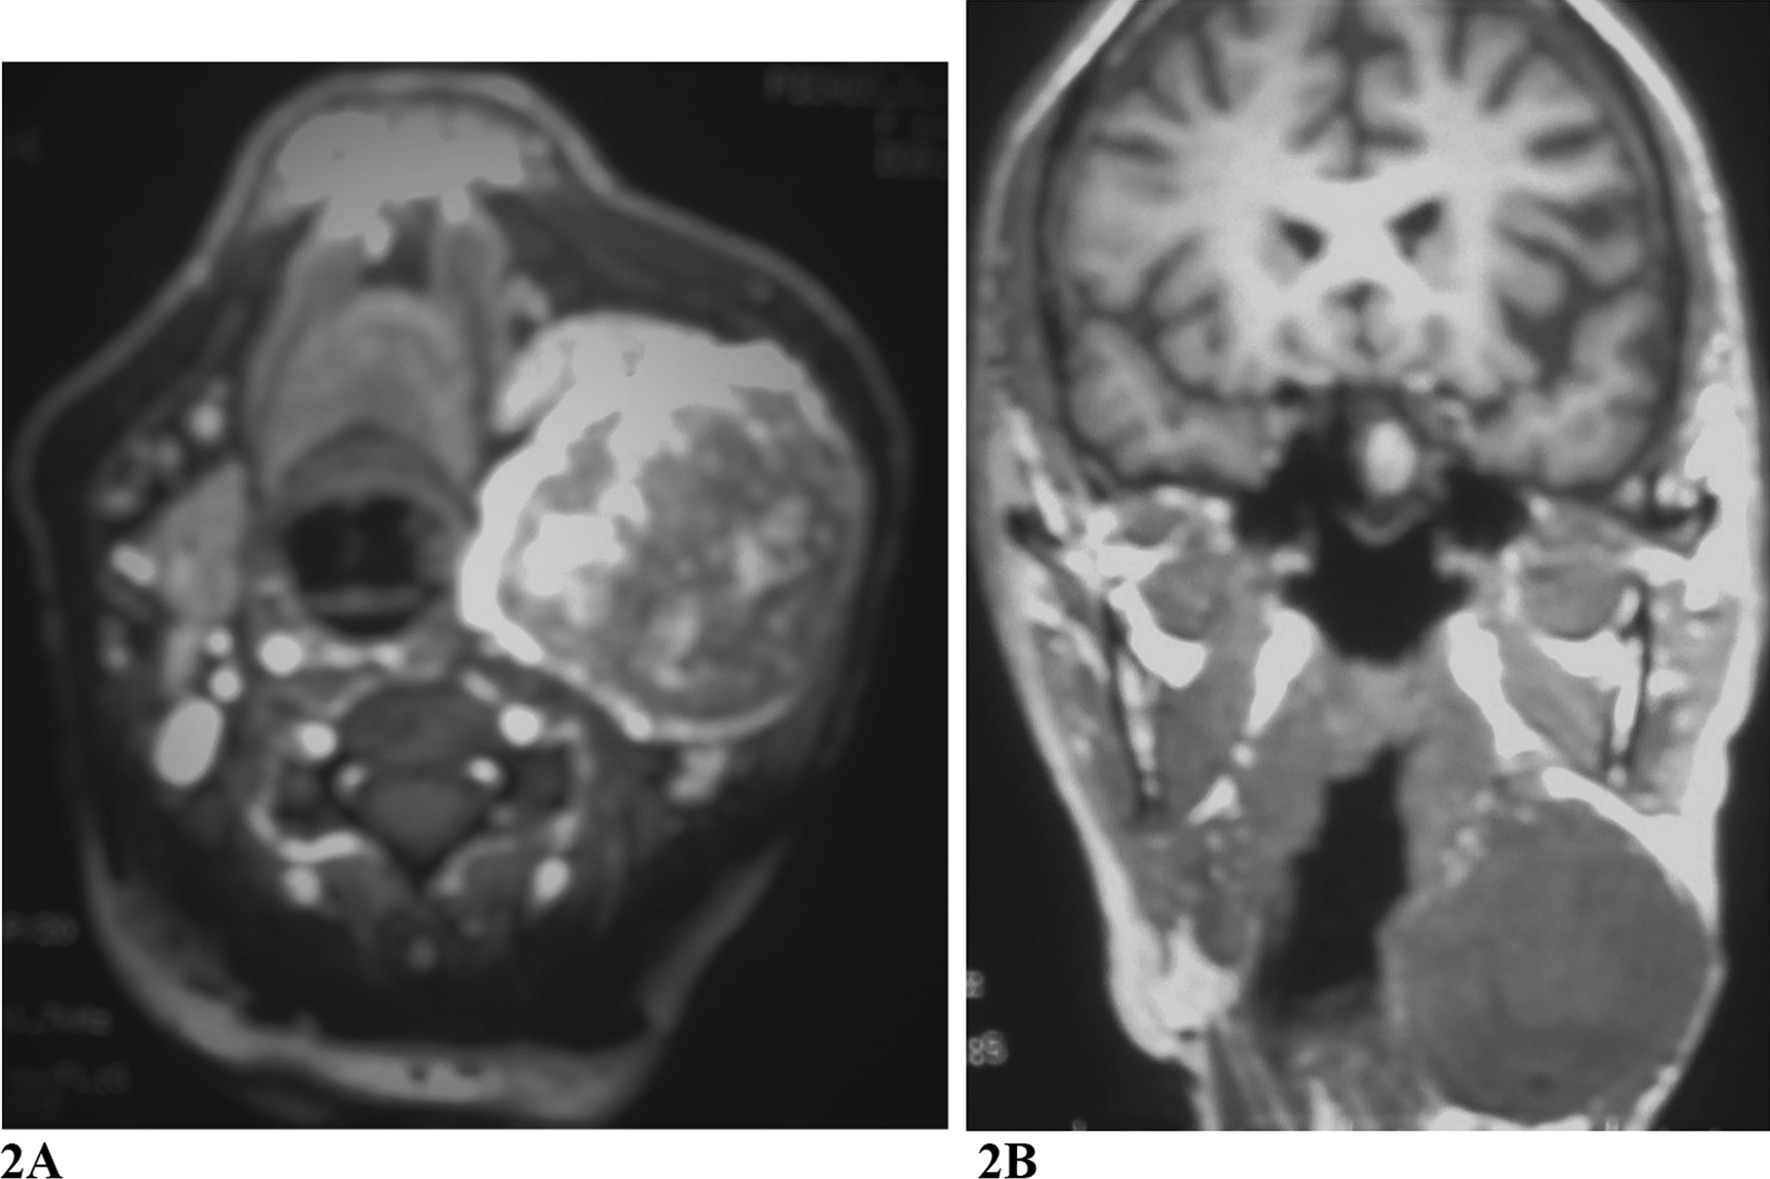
A Angio MRI image showed a 9 cm paratracheal mass on the left cervical side that laterally displaced the sternocleidomastoid muscle and 2 cm to the right side the trachea. B Coronal axial vascular MRI showing the same paratracheal mass on the left cervical side involving the carotid bifurcation
